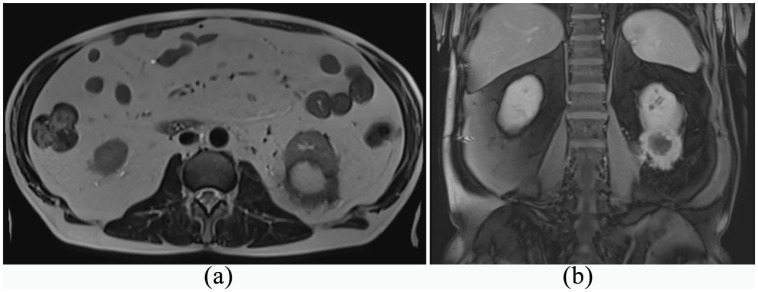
Abdominal MRI: (a) T1-weighted axial image and (b) coronal image.
